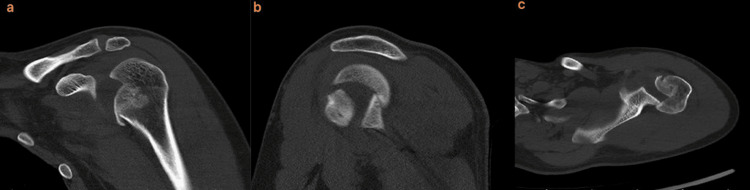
Left shoulder CT coronal (a), sagittal (b) and axial (c) sections taken in the emergency department (Patient 1)
